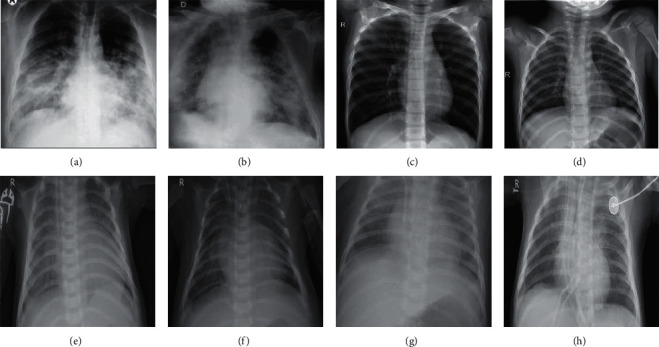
Chest X-ray images. (a) COVID-19, (b) COVID-19, (c) normal, (d) normal, (e) pneumonia—bacteria, (f) pneumonia—bacteria, (g) pneumonia—viral and (h) pneumonia—viral.
